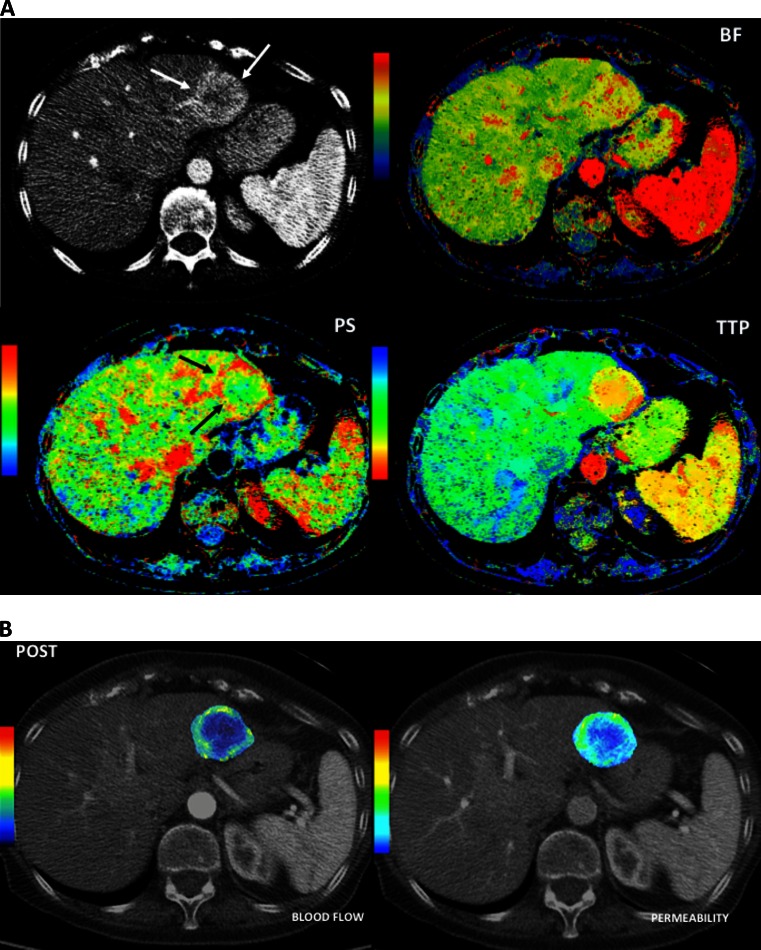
Perfusion CT images in a patient with liver metastases from a neuroendocrine tumor pre- and posttherapy using antiangiogenic drugs. Parametric maps of blood flow and permeability superimposed over conventional CT images. Pretherapy study (a) demonstrated increased mean values of blood flow (115 mL/min/100 g) and permeability (51 mL/min/100 g), mainly at the periphery of the metastatic deposit. Posttherapy exam (b) evidenced a clear tumor response with drastic decrease of the values of both parameters (blood flow = 12 mL/min/100 g and permeability = 12 mL/min/100 g)
